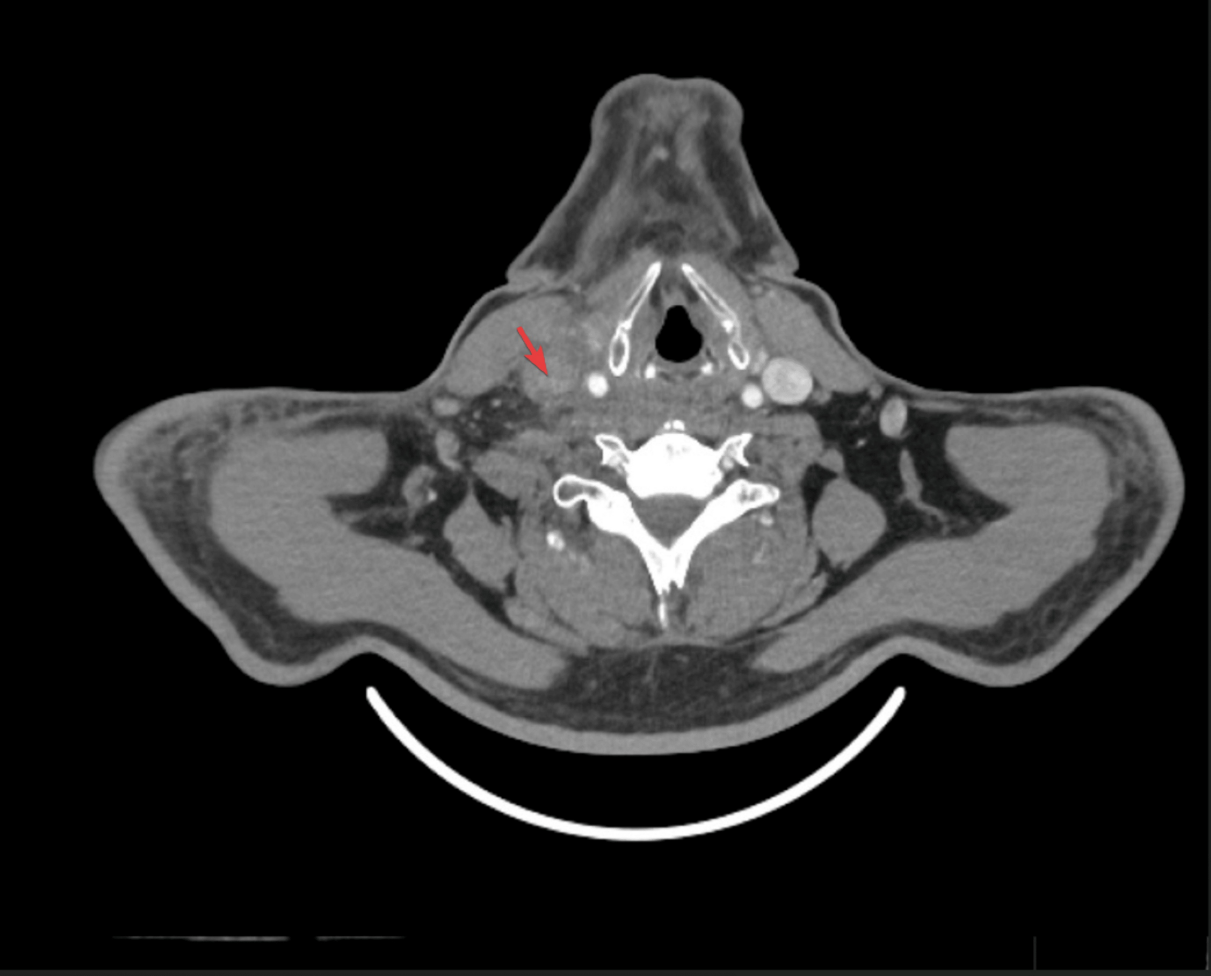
CT neck, chest, abdomen, and pelvis (June 18, 2024) (horizontal view). The arrow indicates a lack of contrast opacification in the right internal jugular vein, with surrounding fat stranding, suspicious of thrombosis.
